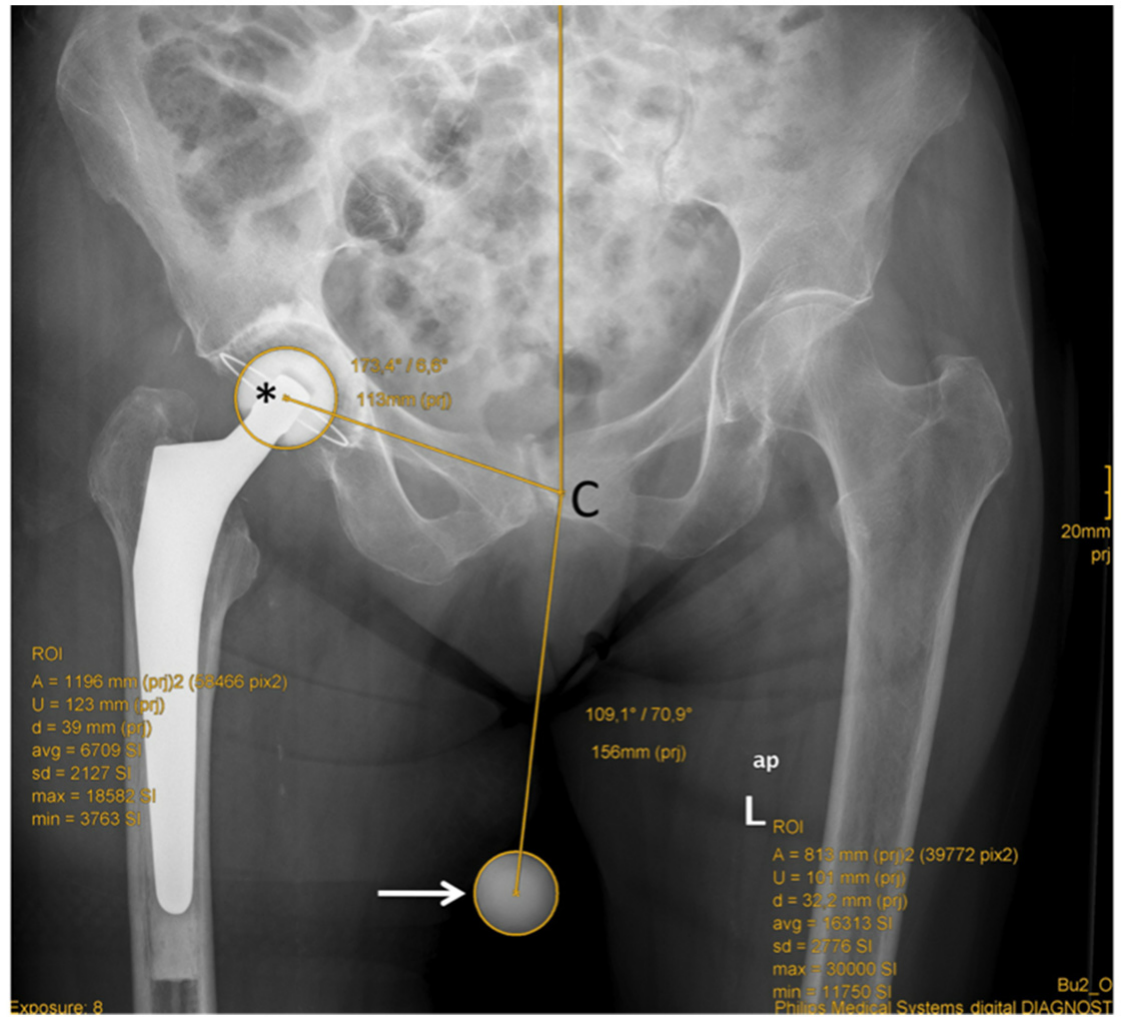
Standing AP radiograph of the pelvis with internal (asterix) and external calibration marker (arrow) and performed measurements: central beam (C); marker diameter; distances of the markers from the central beam; position of the markers.
